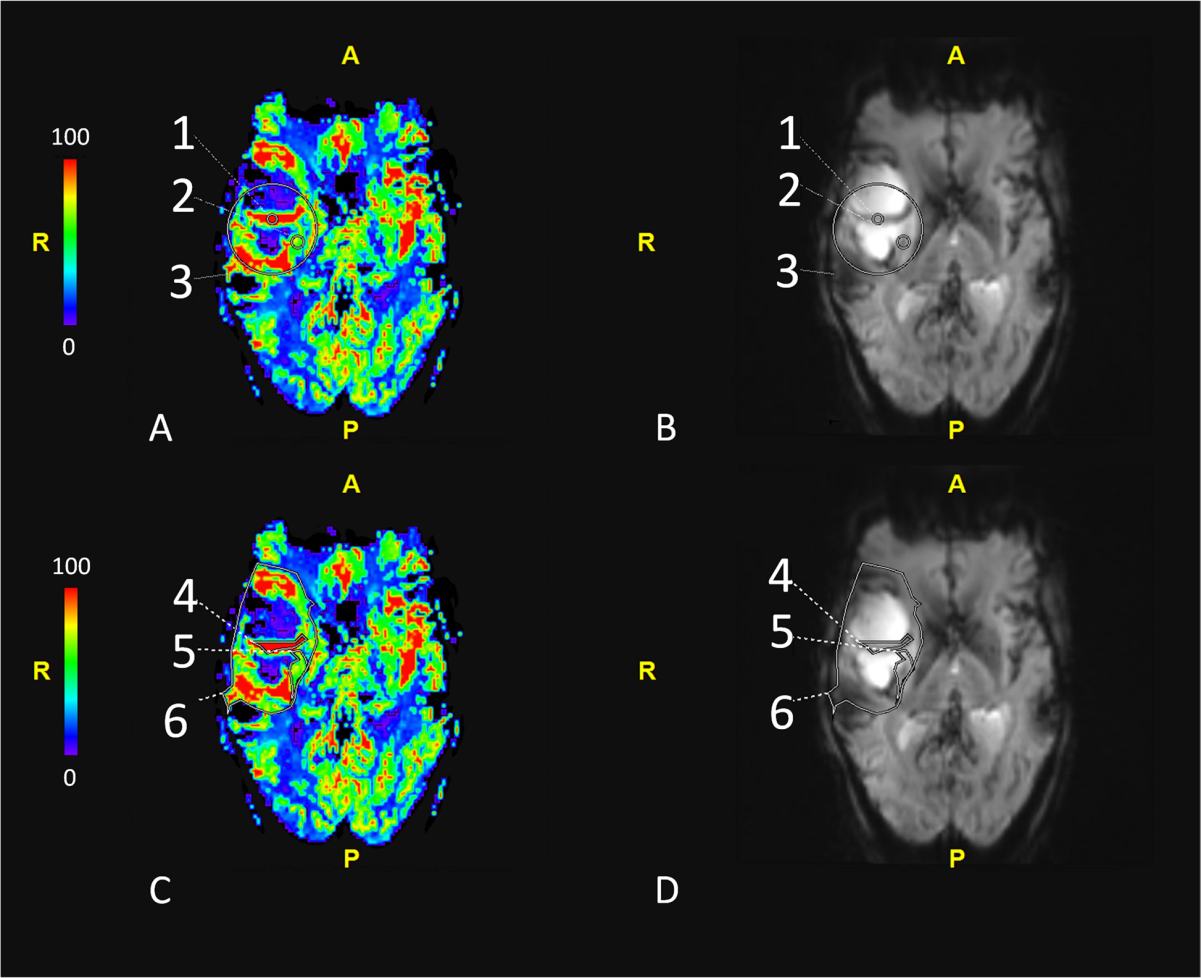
Exemplary images of the different ROIs used for this study. On the left-handed side, the CBV maps are presented (A and C); on the right-handed side, the DSC-MRI source data are presented (B and D). ROI1 = Circular ROI covering the tumor hotspot; ROI2 = Circular ROI covering the peritumoral region; ROI3 = Circular ROI covering the whole tumor region; ROI4 = Polygonal ROI covering the tumor hotspot; ROI5 = Polygonal ROI covering the peritumoral region; ROI6 = Polygonal ROI covering the whole tumor region. DSC-MRI = dynamic susceptibility contrast magnetic resonance imaging; CBV = cerebral blood volume; ROI = Region of interest
